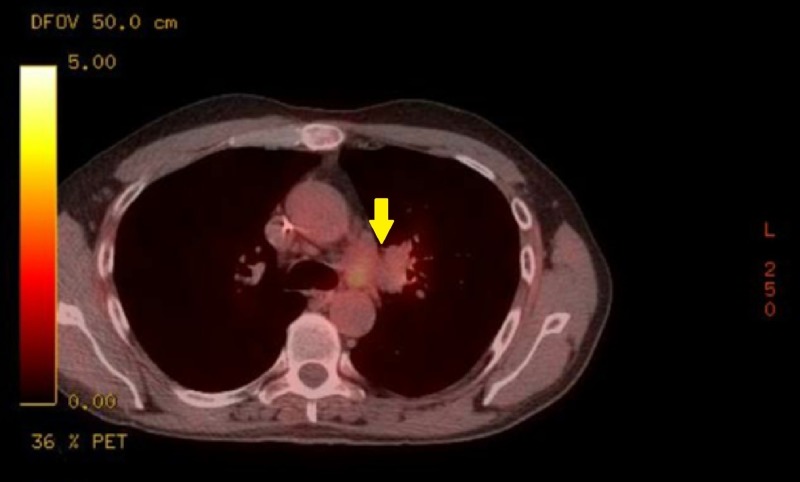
Mediastinal Hypermetabolism Post-Treatment Transverse Positron Emission Tomography (PET) Scan. Fluorodeoxyglucose (FDG) in the left hilum and the left AP window markedly improved with the SUV level 2.2-2.7 compared with the previous 9.3.
